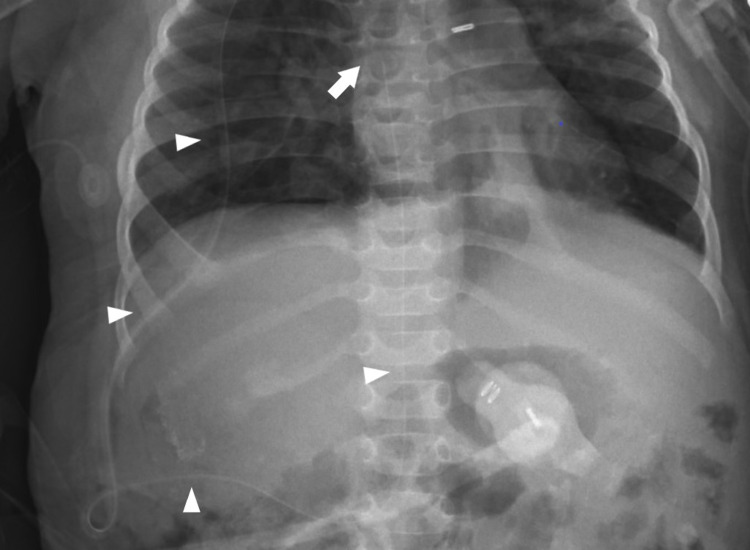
Postoperative radiograph demonstrating distal catheter coursing over the flank and entering IVC (arrowheads) with termination in the right atrium (arrow) IVC: Inferior vena cava
